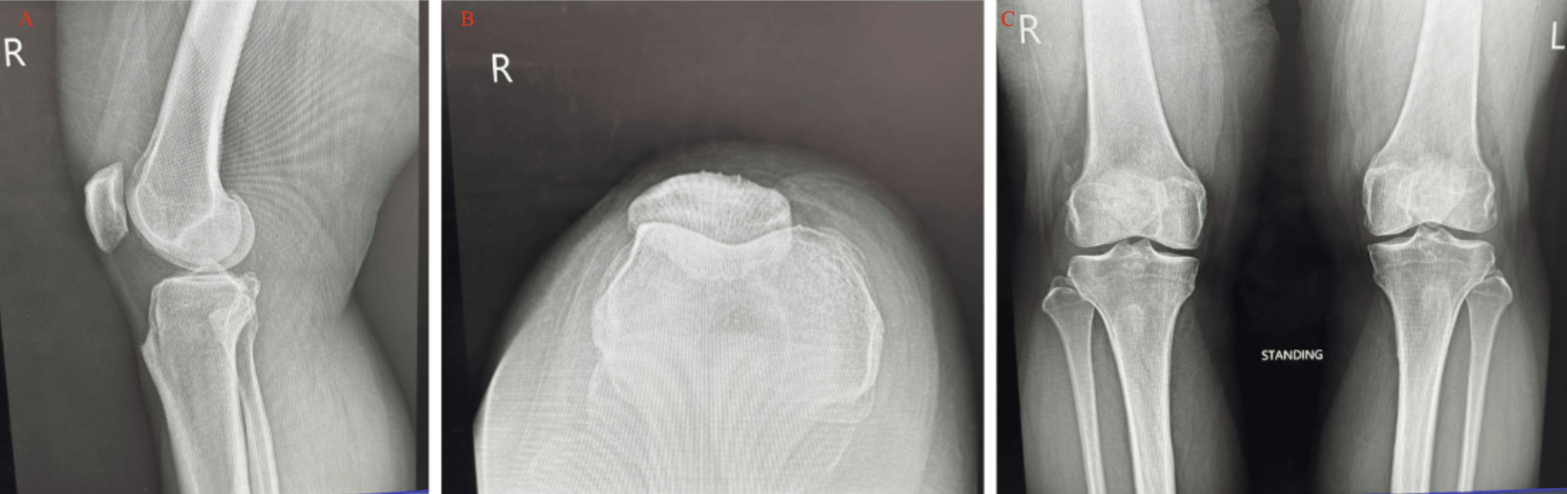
Plain radiographs of the right knee with a lateral view (A), sunrise view (B), and then bilateral anterior-posterior view (C). No joint space degeneration or osseous abnormalities present. Slight patellofemoral syndrome is present, as indicated by the lateral tracking of the patella on the sunrise view.
